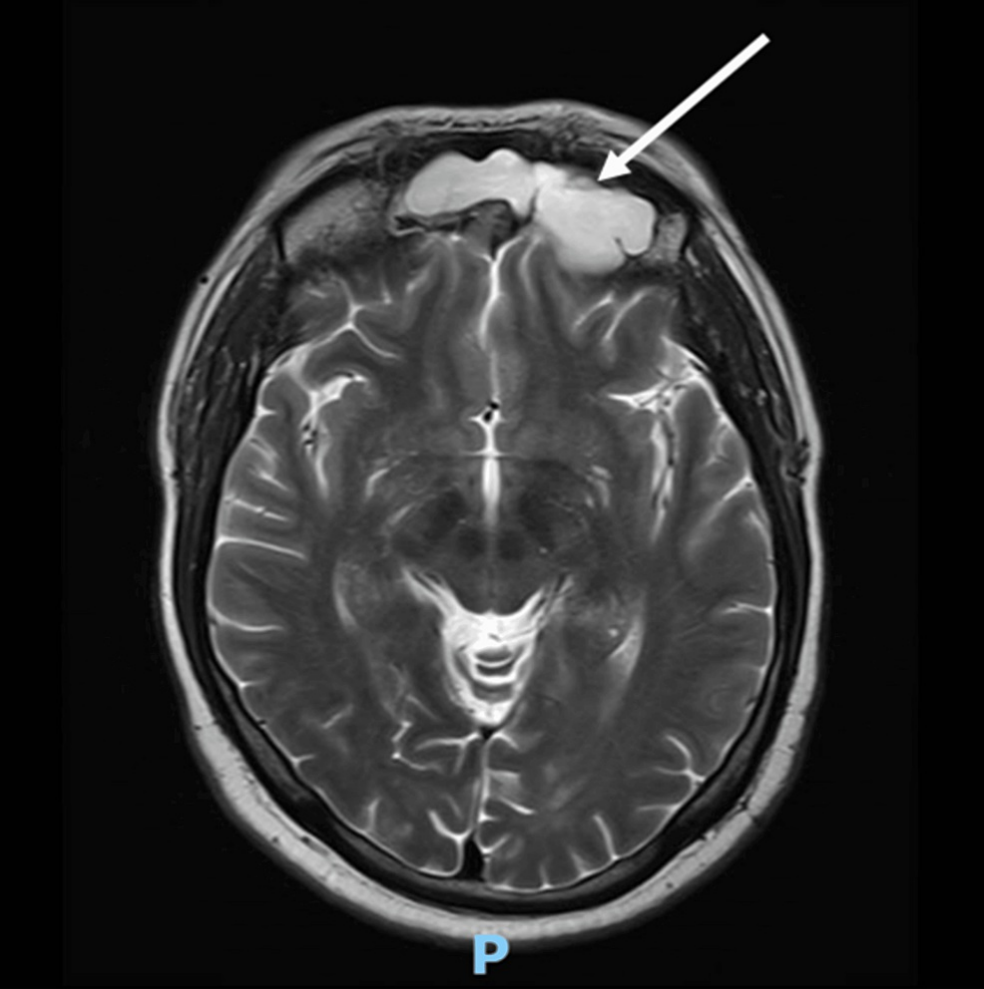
A brain MRI (T1-weighted; without contrast; sagittal plane) shows a soft tissue lesion located in the midline olfactory groove area. Dural surface with extension into anterior frontal dura. MRI: Magnetic resonance imaging
